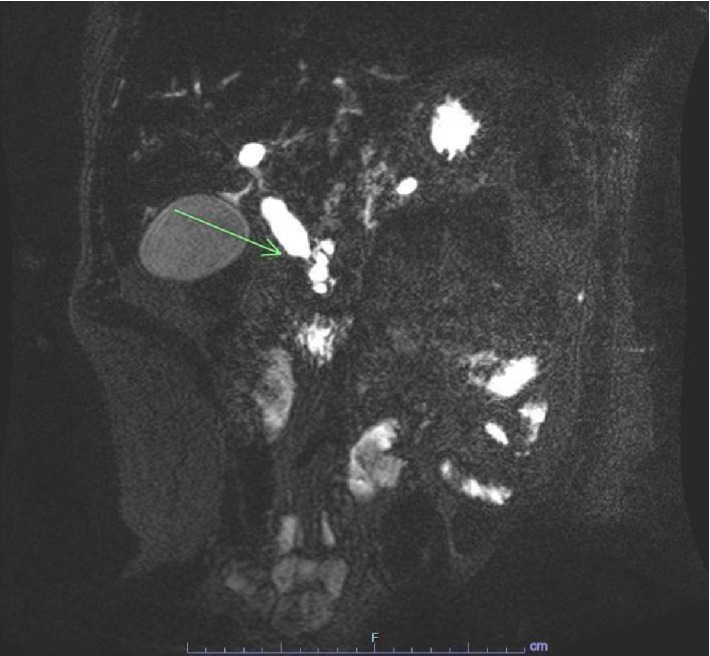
MRI abdomen-enhancing mass in the region of the ampulla, with resulting significant dilation of the biliary tree and the main pancreatic duct.
